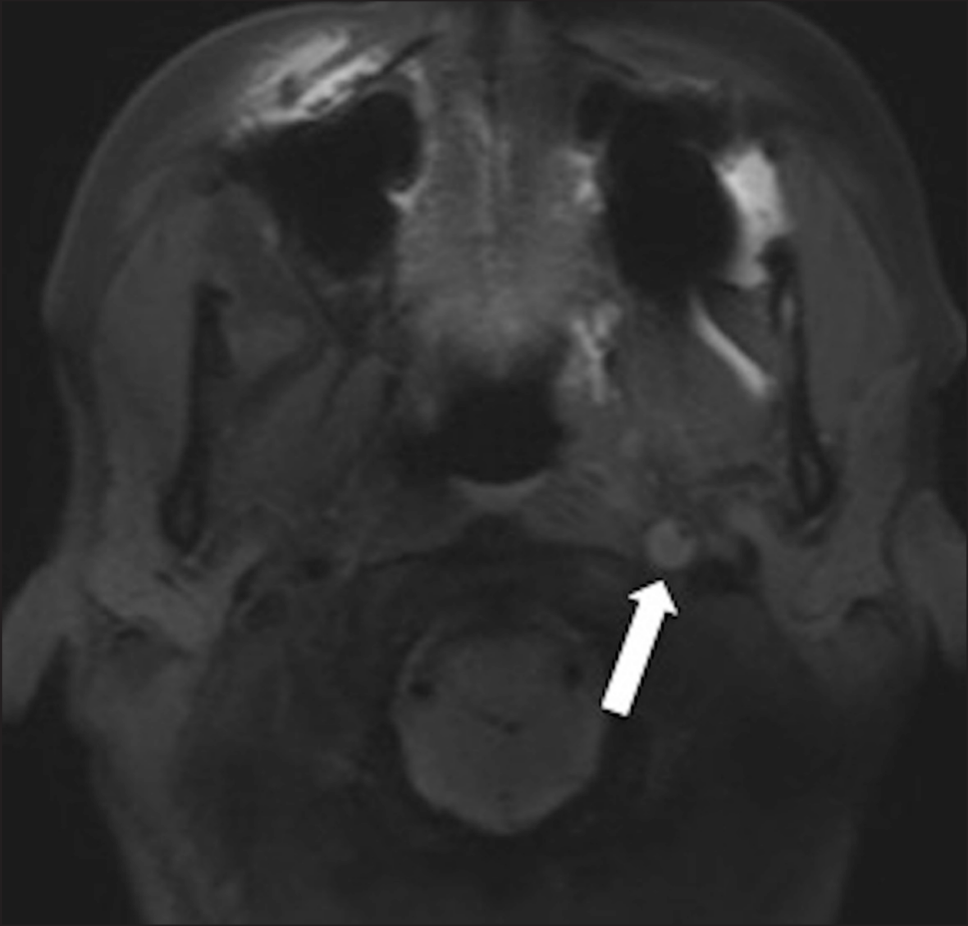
Cervical MRI, volumetric 3D fat suppressed SE T1-weighted sequence allowing to overcome the magnetic susceptibility artifact and to bring out extension of the cervical portion of left internal carotid artery dissection (arrow).
